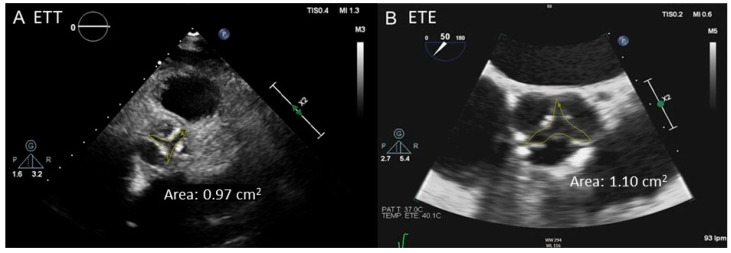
Aortic valve planimetry using TTE (A) vs. TEE (B). A better definition of the free edges of the leaflets with TEE facilitates the tracing; in this case, the valve orifice area moves from 0.97 cm^2^ ((A) severe stenosis) to 1.10 cm^2^ ((B) moderate stenosis). TEE: transesophageal echocardiography. TTE: transthoracic echocardiography.
